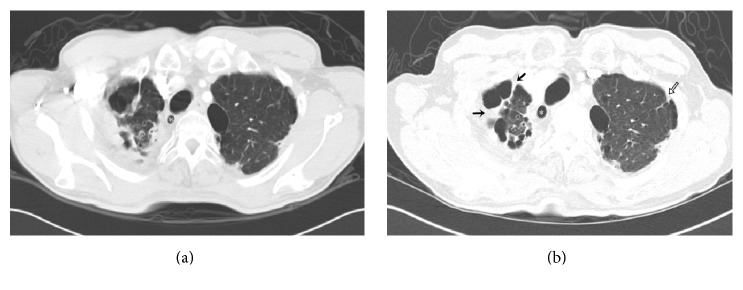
(a) Axial CT scan shows a paratracheal air cyst (*∗*) and fibrosis with volume decrease in a right upper lung and subpleural fibrosis in a left upper lung. (b) CT scan obtained 32 months later shows an increase in PAC size. In addition, fibrosis had thickened (arrow) and the volume of the right upper lobe has reduced. Subpleural fibrosis is more prominent in the left upper lung (black line arrow). The total fibrosis score of both upper lungs increased from 9 to 12 points over the 32 months.
